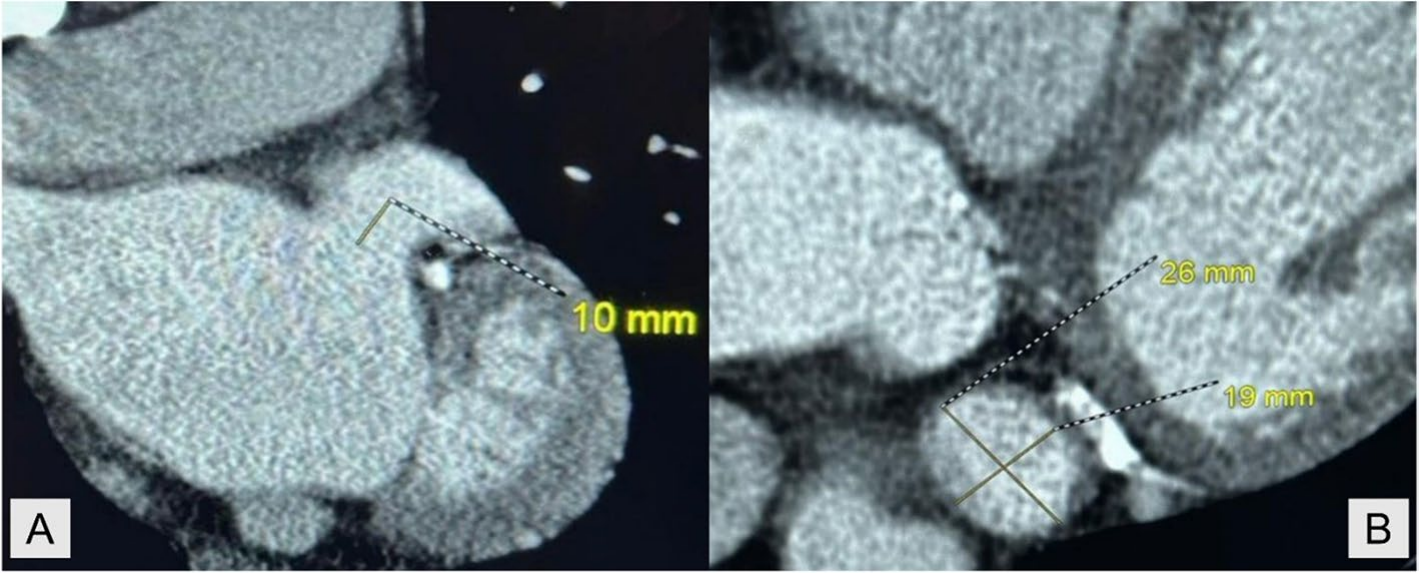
Computed tomography measurement of left atrial appendage (LAA) dimensions. A Coronal projection of the LAA with maximal craniocaudal depth from the LAA ostium. B Axial projection of the LAA with maximal landing zone diameter
